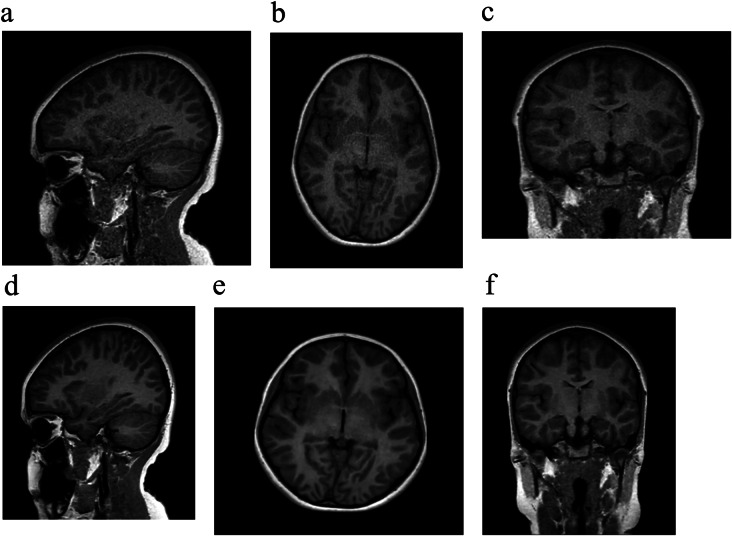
(a-f): Sagittal 3D T1-weighted SPGR CS = 8 with axial and coronal reformats in the same patient as Fig. 1 was performed with standard (a-c) and AI (d-f) reconstruction with improved overall image quality on the AI sequences marked by increased SNR and image sharpness
